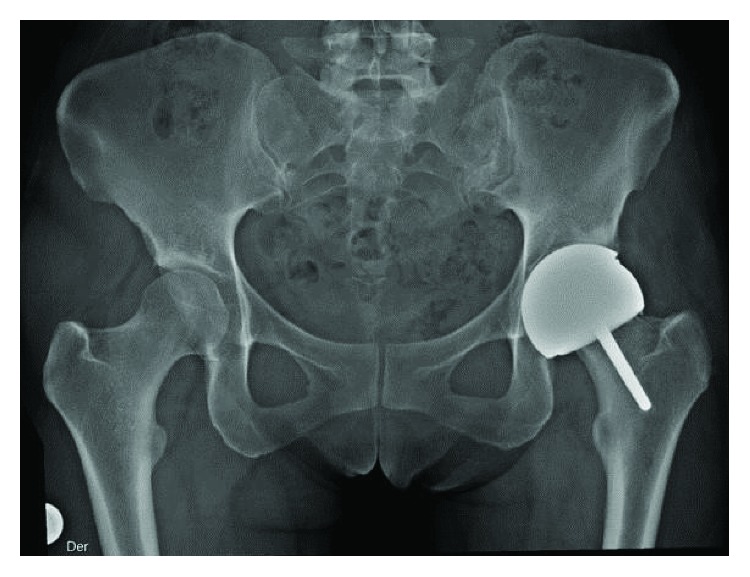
Postoperative AP radiograph showing the left hip's resurfacing arthroplasty. Femoral head was 46 mm. Acetabular cup was 52 mm and was placed too vertical; malposition of the components is a risk factor for pseudotumour [8].
